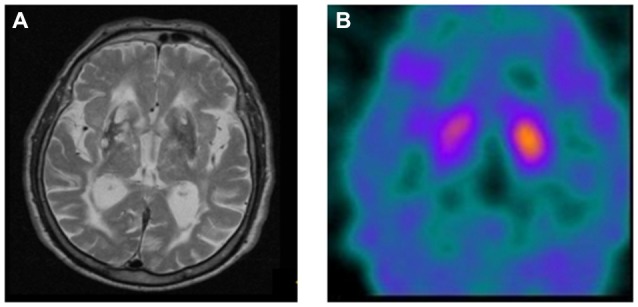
MRI and ^123^I-FP-CIT SPECT Images from a representative patient to show relation existing between abnormal MRI and uptake on ^123^I-FP-CIT SPECT. (A) MRI T2-potentiated showing diffuse cerebral atrophy with increased size and depth of cerebral grooves and ventricular supratentorial enlargement. Multiple lacunar chronic infarctions, some of those were hemeorrhagic in the lenticular and head of caudate nuclei. Chronic periventricular ischemia leucoencephalopaty and focal hyperintensities in parietal frontal and temporal bilateral white matter WSTS = 1.6. (B)
